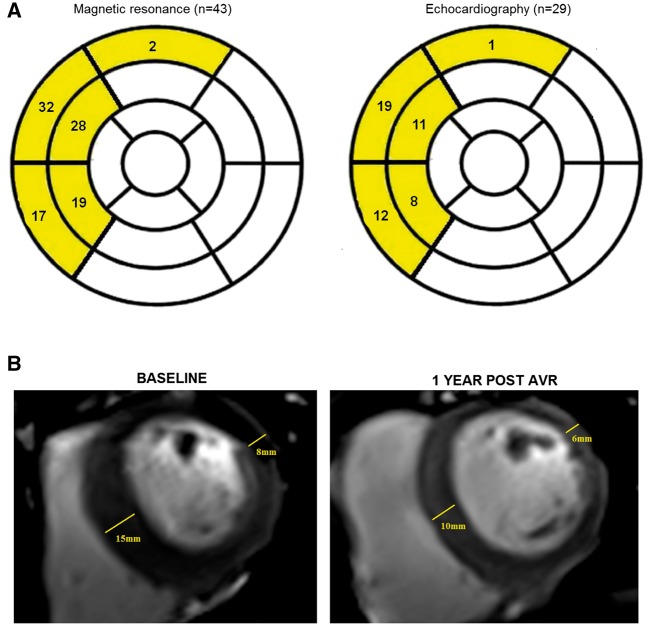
Prevalence, distribution, and resolution after aortic valve replacement of asymmetric wall thickening. (*A*) Seventeen segment model of the LV demonstrating the site of asymmetric wall thickening as detected by both magnetic resonance and echocardiography. Magnetic resonance was more sensitive in detecting asymmetric wall thickening (43 cases) than echocardiography (29 cases). On both modalities, asymmetric wall thickening was almost universally confined to the basal and mid-cavity segments of the septum. (*B*) Patient with asymmetric wall thickening at baseline, which resolved when magnetic resonance was repeated 1 year after aortic valve replacement.
